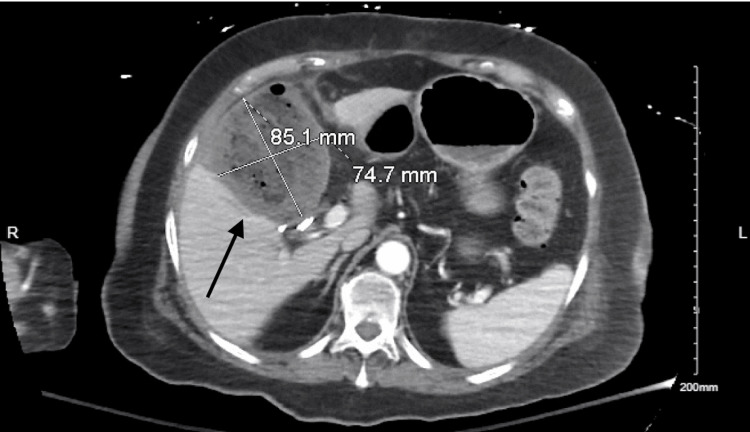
Contrast-enhanced CT scan revealing a large biloma in the subhepatic space
